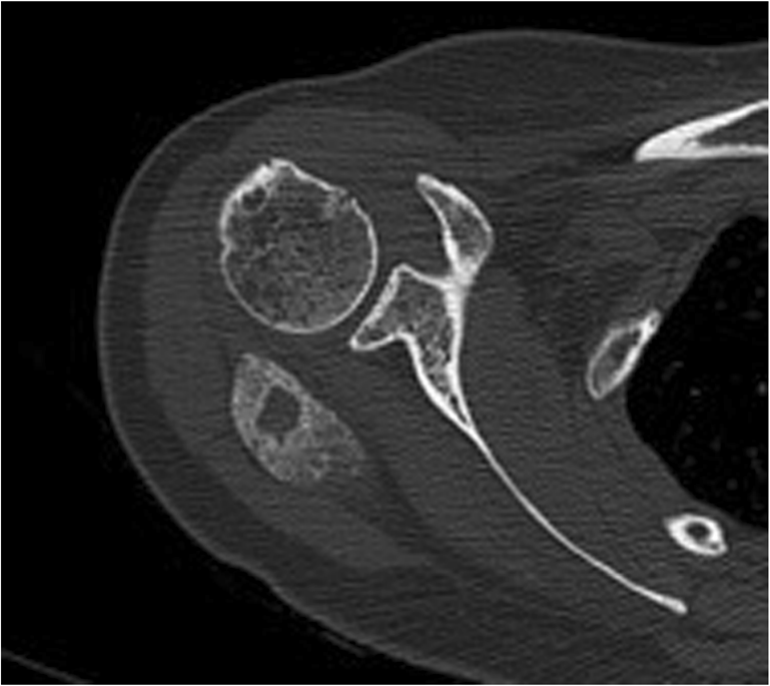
Ossification. Axial CT image of myositis ossificans in the infraspinatus muscle shows a cortical and trabecular mineralisation pattern with a zonal distribution (absence of mineralisation centrally due to fatty bone marrow)
